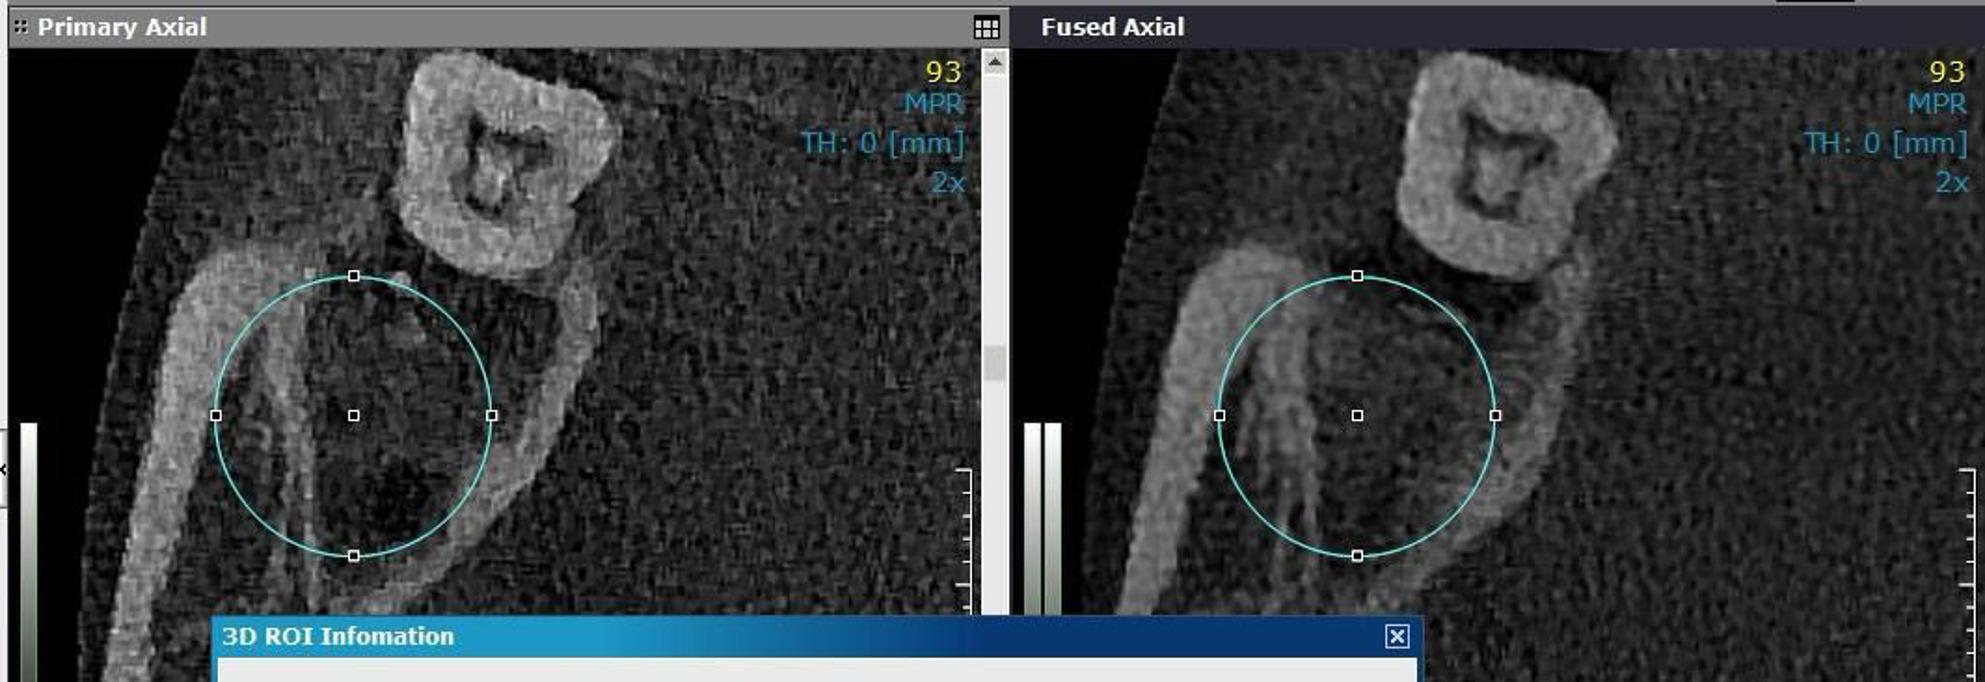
CBCT axial images demonstrating bone density analysis at the extraction site. A three-dimensional region of interest (ROI) was defined within the socket, and the mean gray value was calculated using the imaging software
